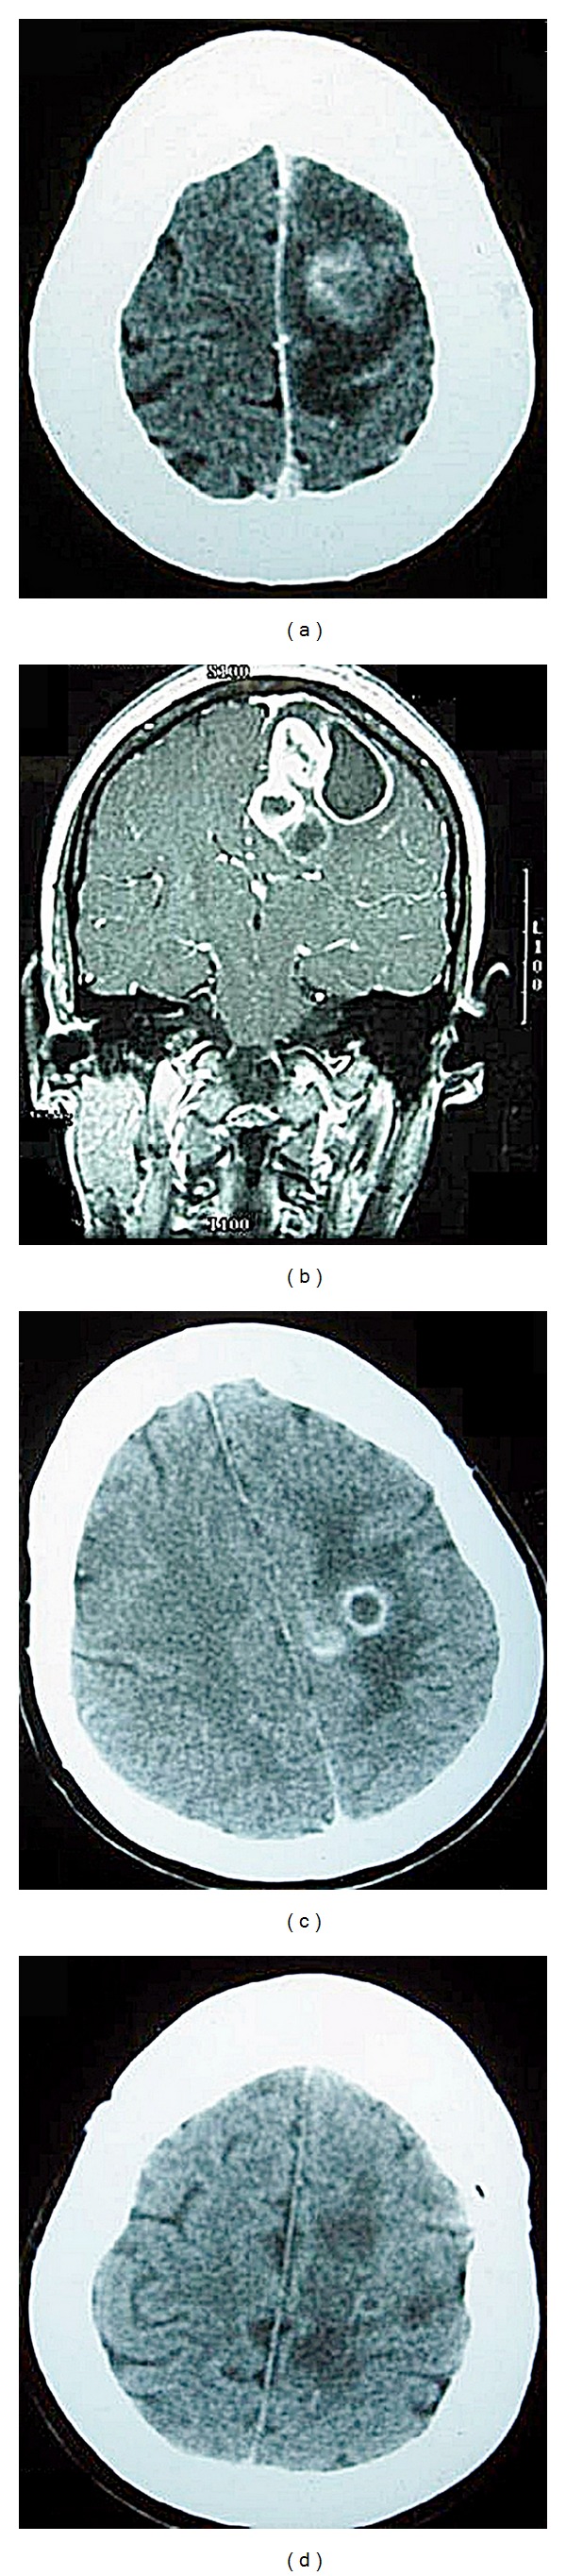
(a) Brain computed tomography (CT) showed ring enhanced nodules with extensive perifocal edema over left frontoparietal region on February 16, 2002. (b) Coronal enhanced T1-weighted magnetic resonance image (MRI) on February 26, 2002, showed multiple conglomerated lesions over left posterior frontal lobe with enhancement. (c) Brain CT on April 8, 2002, and (d) April 15, 2002, showed the gradual regression of the abscesses.
